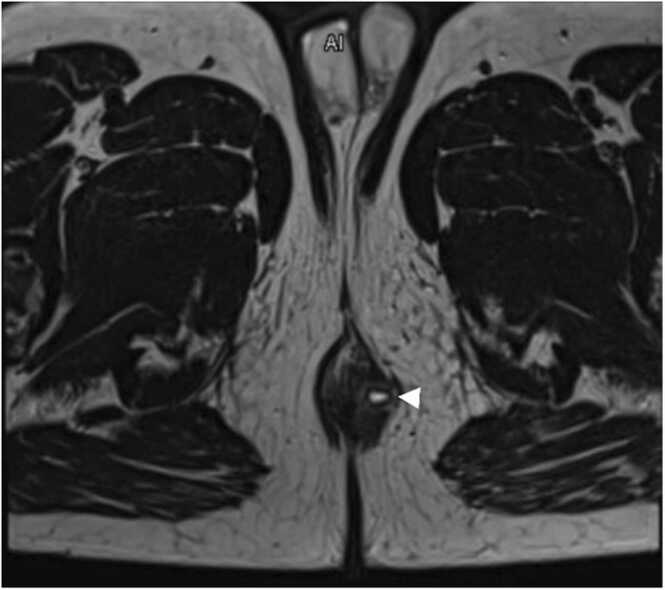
T2 weighted MRI image of the perineum area: Shows hyperintense lesion (arrowhead) on the left side of anal canal, suggestive of left-sided perianal fistula.
